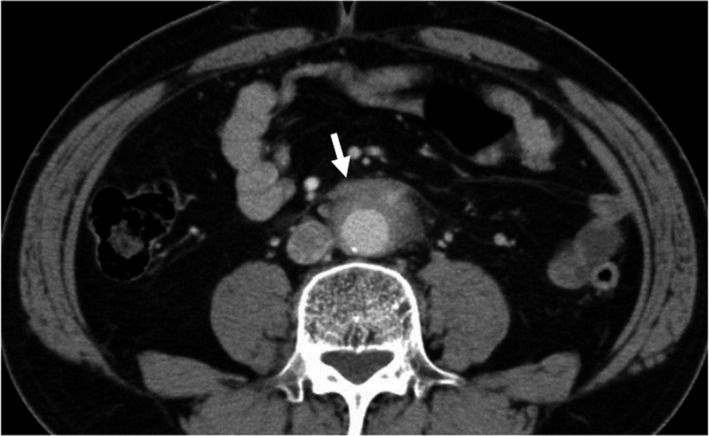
CT image of IgG4‐related retroperitoneal fibrosis. Soft‐tissue mass surrounding the abdominal aorta is recognized (arrow)
